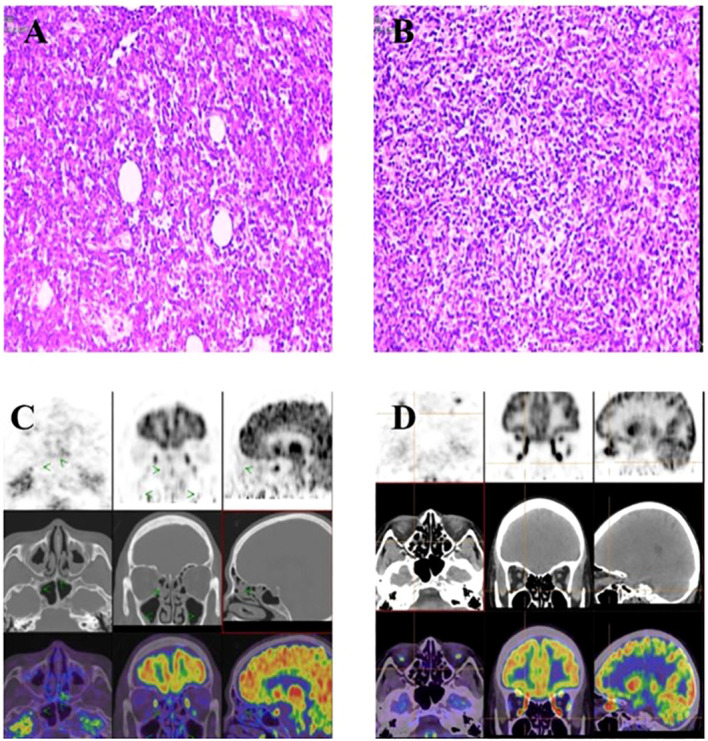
Lymph node case images of the patient and comparative PET/CT scans before and after treatment. (A, B) show the pathological results of the cervical lymph node biopsy (2024.11.1); (C) shows PET/CT imaging (2025.1.13); (D) shows PET/CT imaging after 12weeks treatment (2025.3.27).
